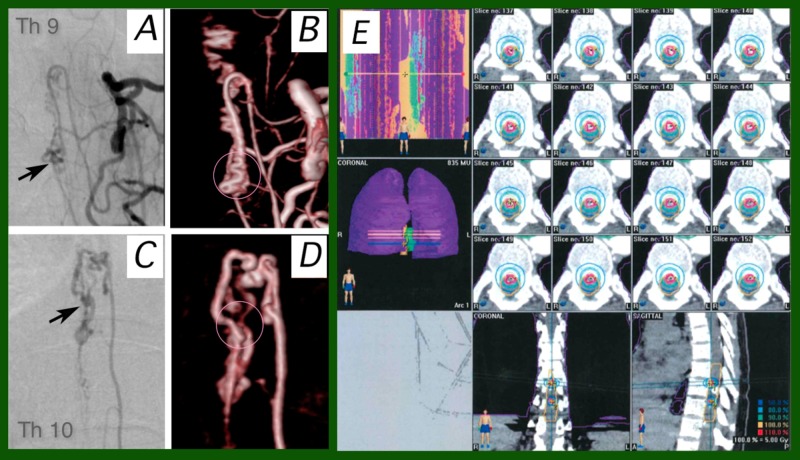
Case 4 Frontal views of left spinal angiography (4A, C) and CT angiography (4B, D) five years after embolization following hemorrhage. Two portions of the residual nidus (arrows) were identified at the levels of T9 (4A, B) and T10 (4C, D). 4E: dose-planning of stereotactic radiotherapy (SRT) on BrainSCAN workstation. Two-arc SRT plan, with one arc each for each portion of the nidus, was adopted. CT: computed tomography; T: thoracic
